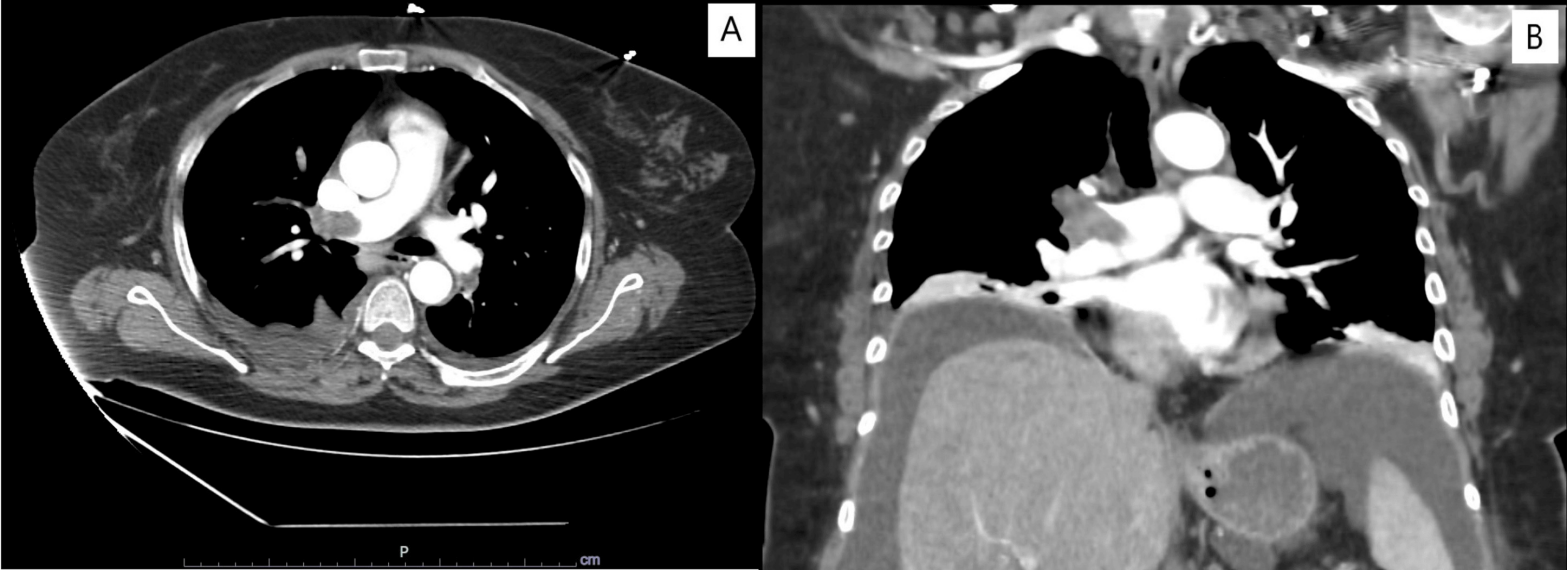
Initial thoracic CTPA showing bilateral PE (panel A – axial view, panel B – coronal view) CTPA: computed tomography pulmonary angiography, PE: pulmonary embolism
